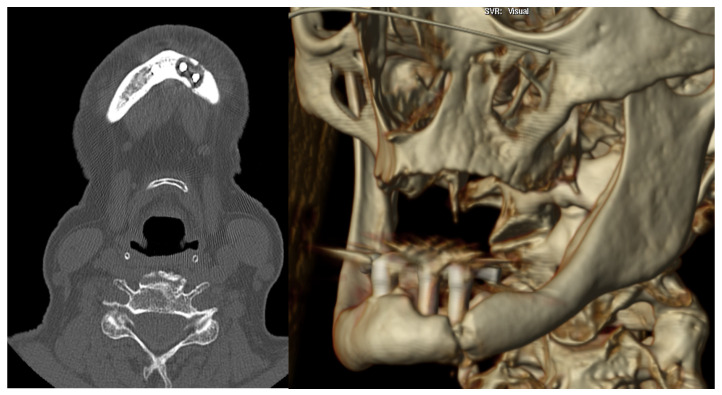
CT scan image: left mandibular parasymphisis fracture secondary to peri-implantitis.
